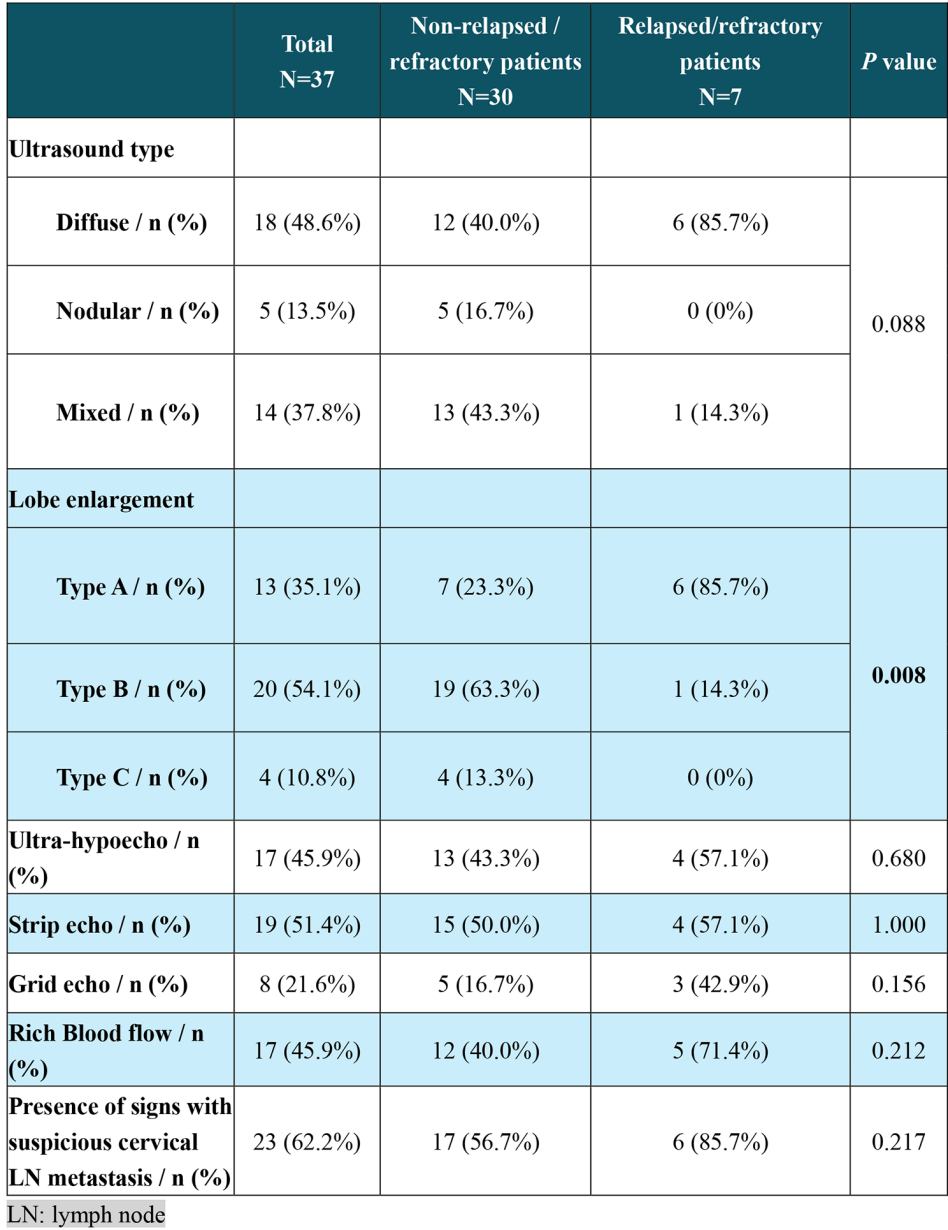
Patient sonographic features
